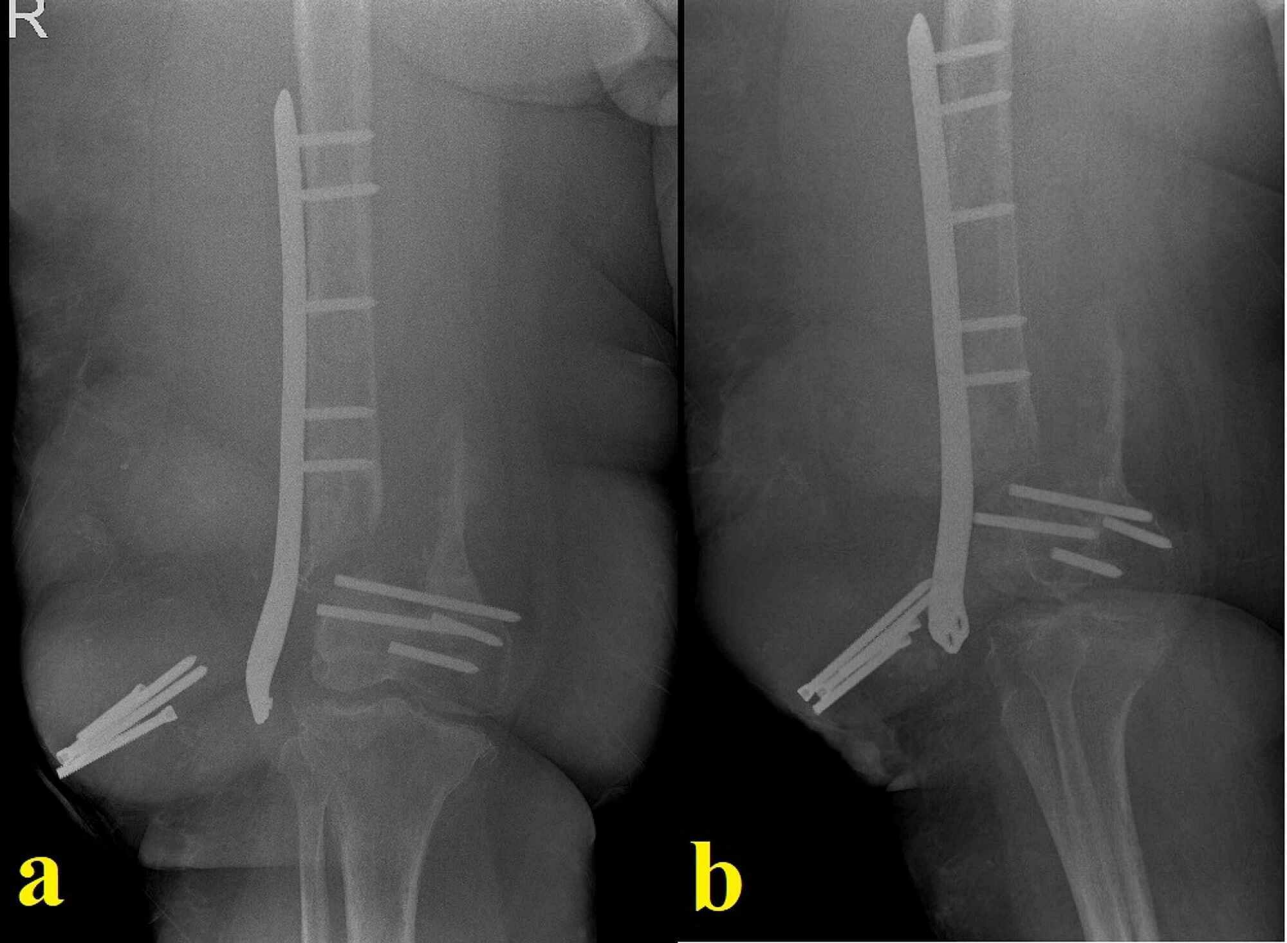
Radiograph at six months postoperatively The image shows failure at the fracture site with no signs of callus, resorption, thinning of the cortexes, and multiple failed distal screws
